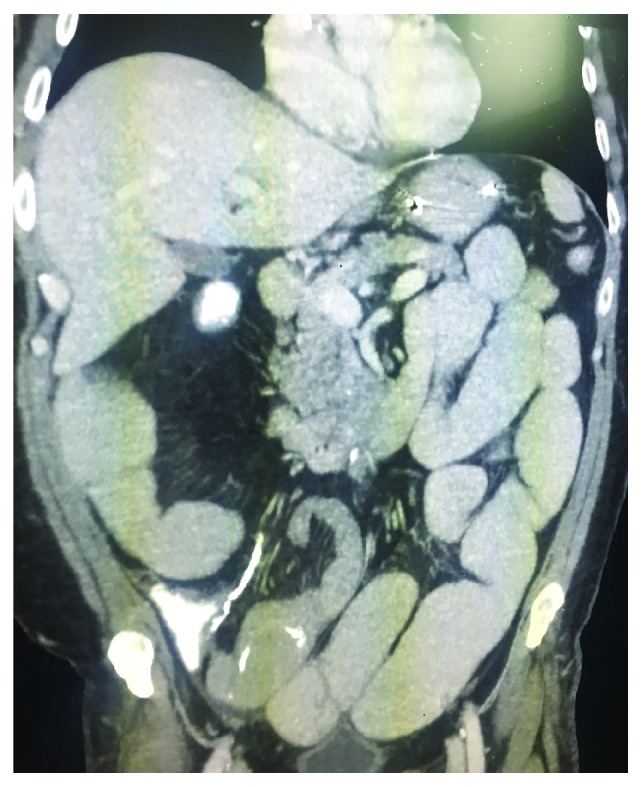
CT imaging showing narrowing of the ileum distal to the previous anastomosis.
